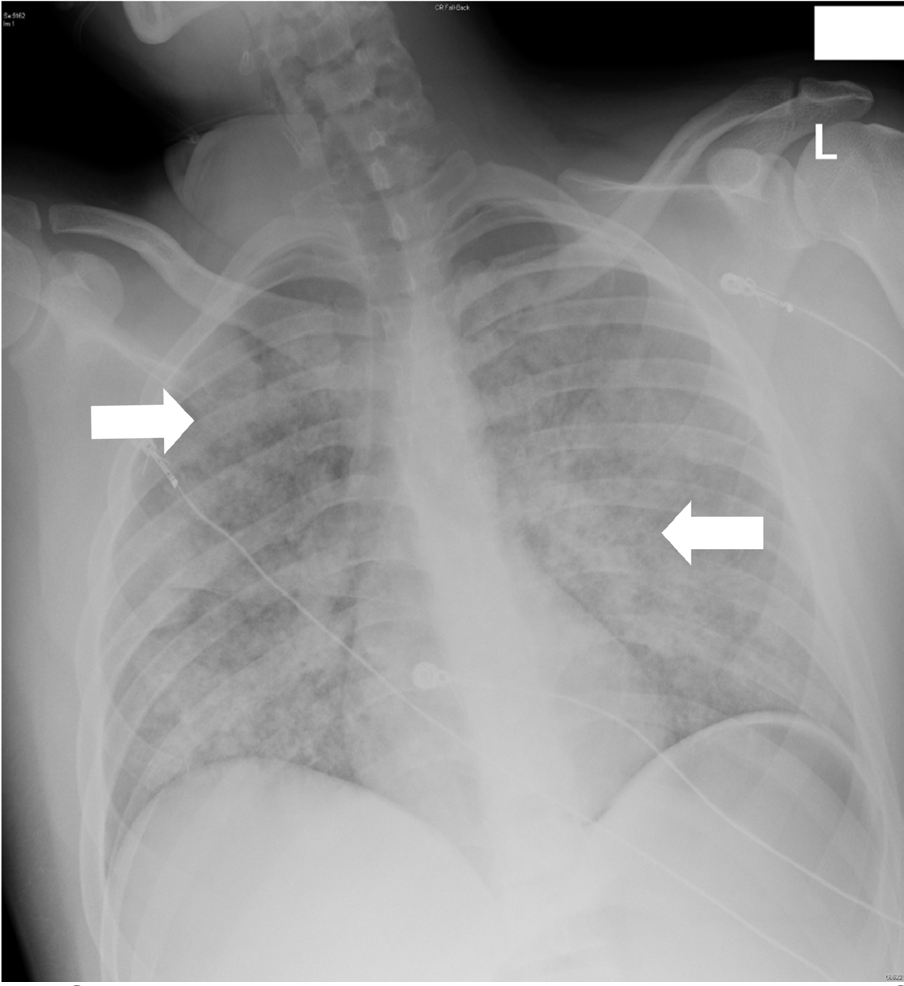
Chest radiograph at initial presentation showing diffuse pulmonary infiltrates. White arrows showing infiltrates.
